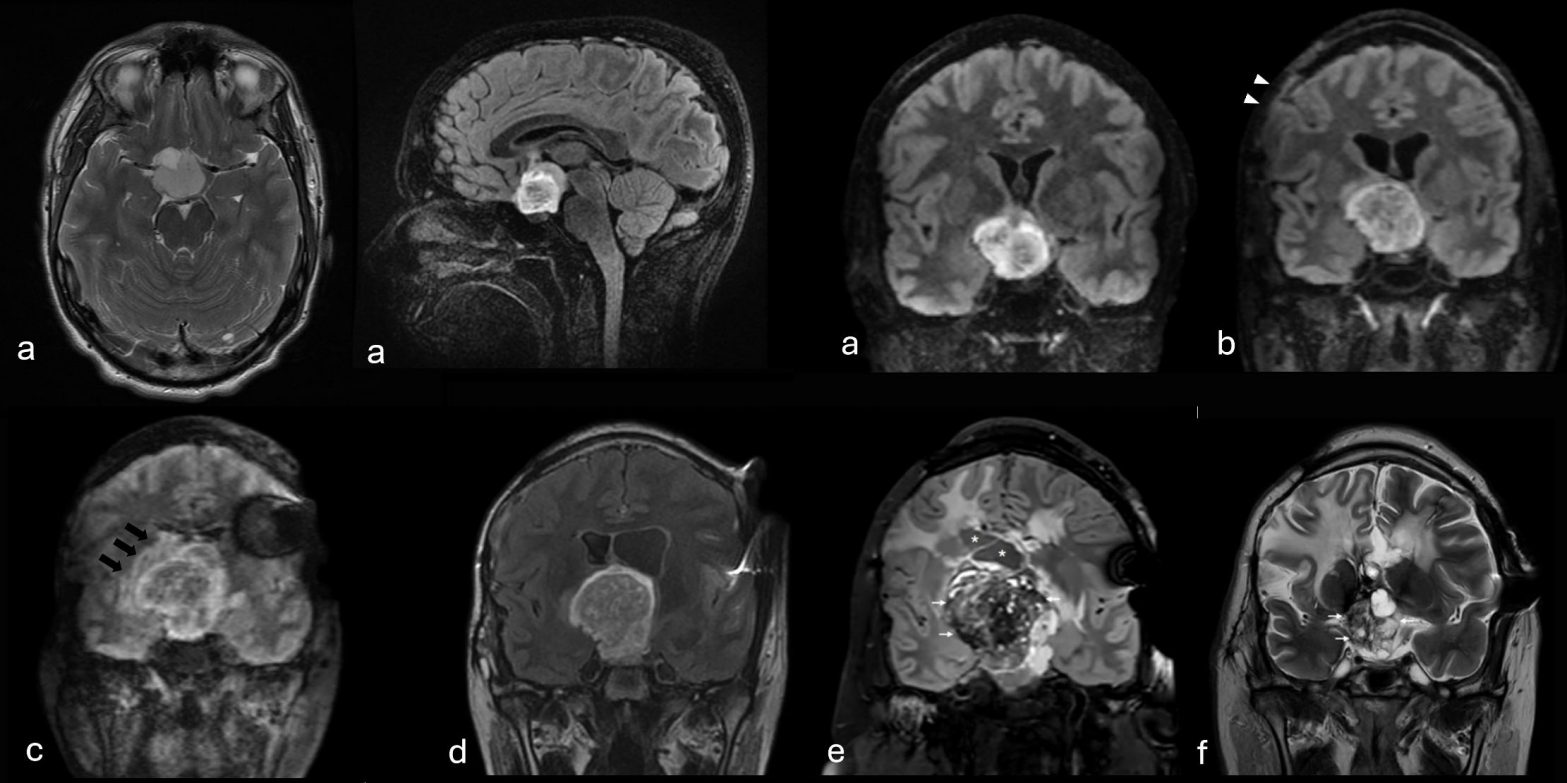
T2−weighted FLAIR MR images depicting the 7−year longitudinal evolution of Patient 2 with a solid–cystic optic pathway glioma. (a) At presentation, the lesion shown is predominantly solid, centered in the suprasellar cistern, involving the optic chiasm and mildly indenting the right hippocampal uncus (axial, sagittal and coronal images, respectively). (b) After two partial resections (2019), postoperative changes are evident (arrowheads), with increased mass effect and ventriculomegaly. (c) Following completion of vinblastine therapy, the tumor shows marked interval growth with increased perilesional edema (black arrows). Susceptibility artefacts from the left parietal VP shunt valve are visible. (d) Tumor status at the end of trametinib therapy (December, 2020). (e) On subsequent follow−up immediately prior to initiation of proton therapy (March, 2023), the tumor is markedly enlarged, with numerous cystic components and pronounced perilesional edema. (f) Coronal T2 TSE images one year later. The solid portion (arrows) showed an important decrease in size and mass effect, and the cystic portions (asterisks) at its most cranial margin were almost completely reduced.
